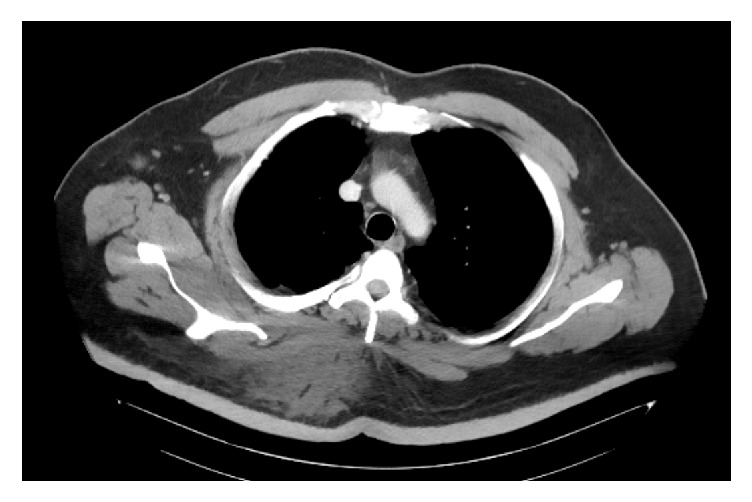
CT scan of the chest showing a large area of subcutaneous tissue density of right upper back.
